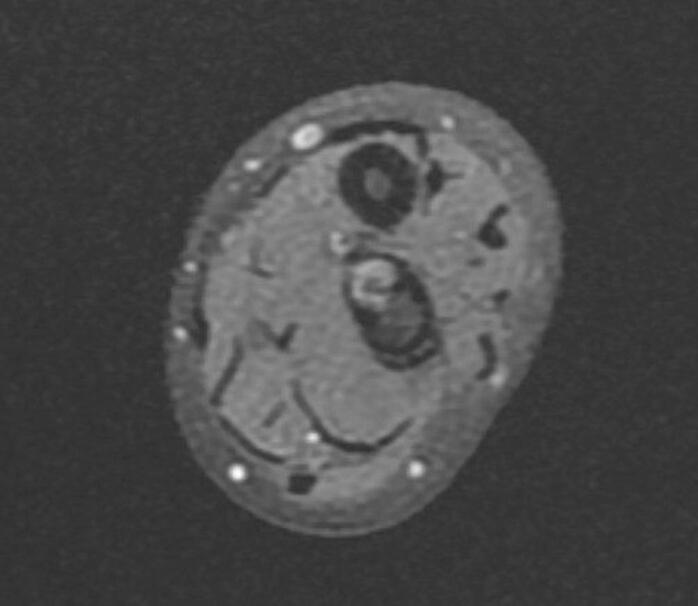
Preoperative MRI indicates the FCD at the distal ulna (axial view).
